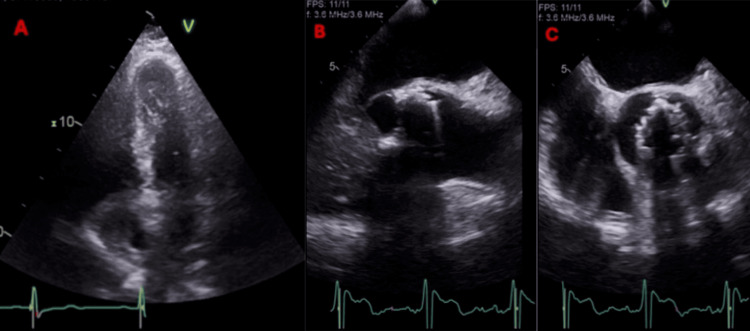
Echocardiographic images showing no evidence of infective endocarditis. (A) Four-chamber view on transthoracic echocardiography (TTE).
